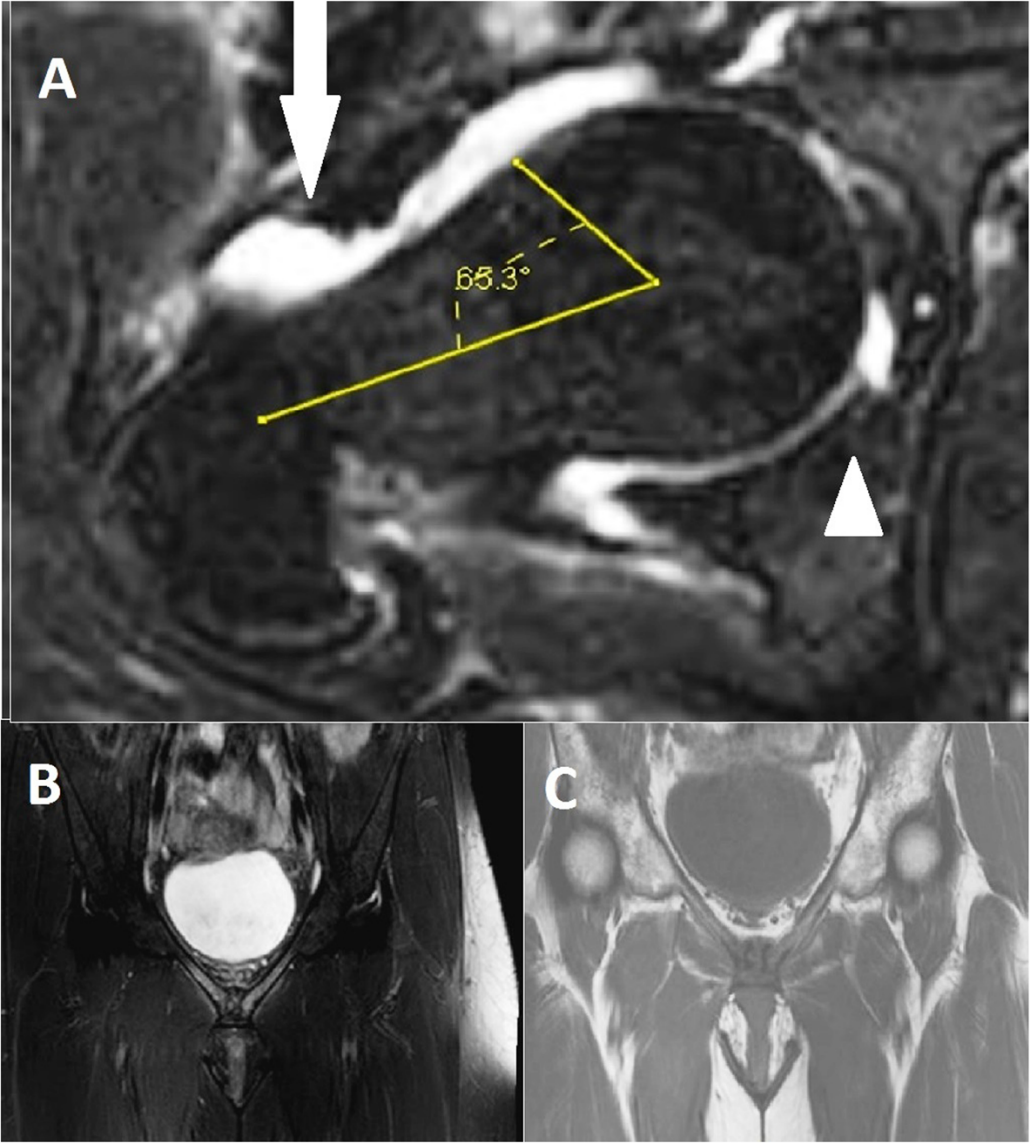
Forty-five-year-old female with chronic hip pain and diagnosed femoroacetabular impingement. a Axial fat-saturated T2-weighted MR image shows an increased alpha angle (65.3°), paralabral cyst (solid arrow), and labral tear (arrow head). b Coronal T1-weighted MR image showing acute-on-chronic osteitis pubis with osseous irregularity, degenerative changes, and hypointensity on both sides of the symphysis pubis. c Coronal fat-saturated T2-weighted image of MR showing diffuse bone marrow and soft tissue edema
